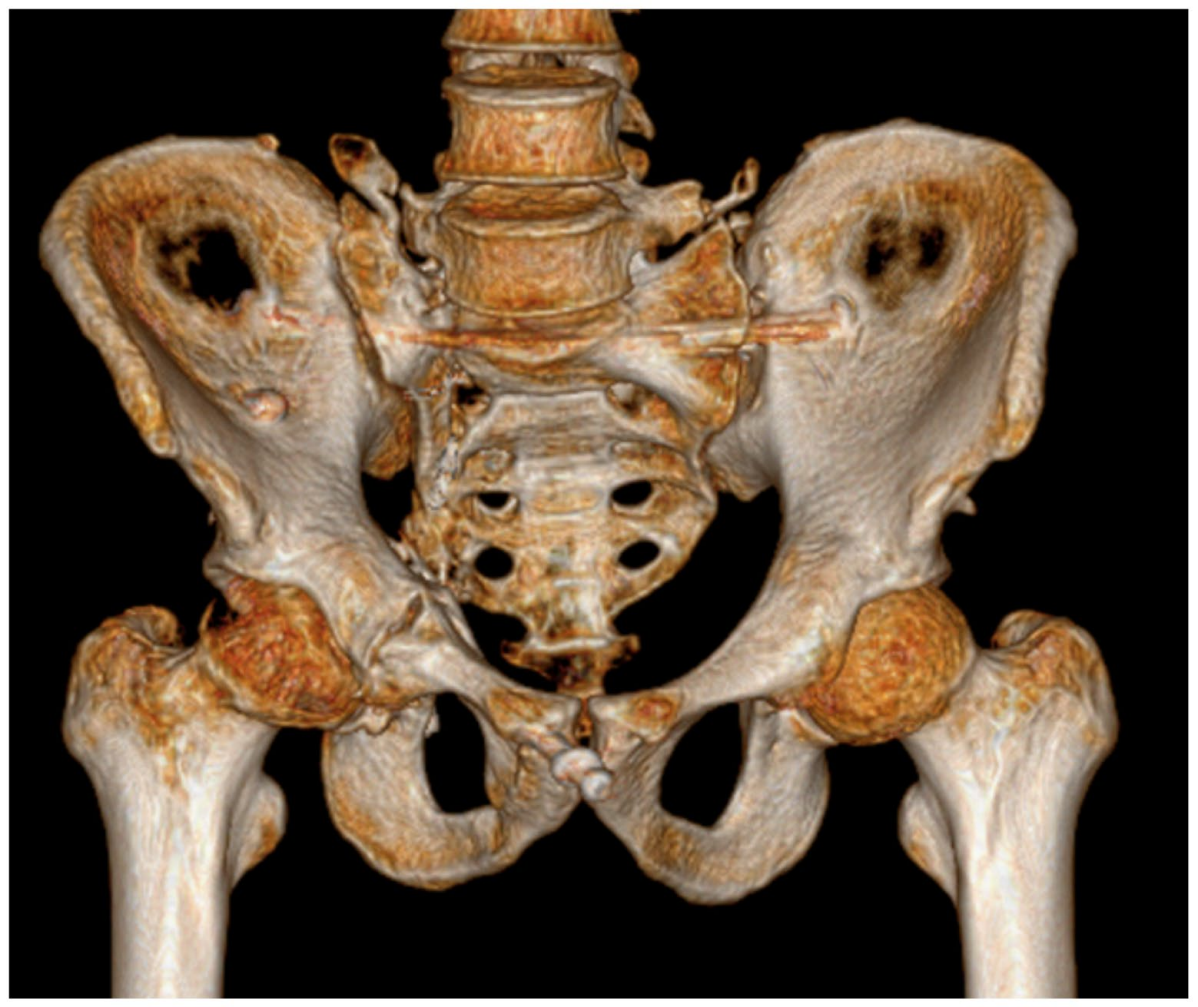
Two years postoperatively, pelvic three-dimensional computed tomography demonstrates good healing of the pelvic and acetabular fractures.
